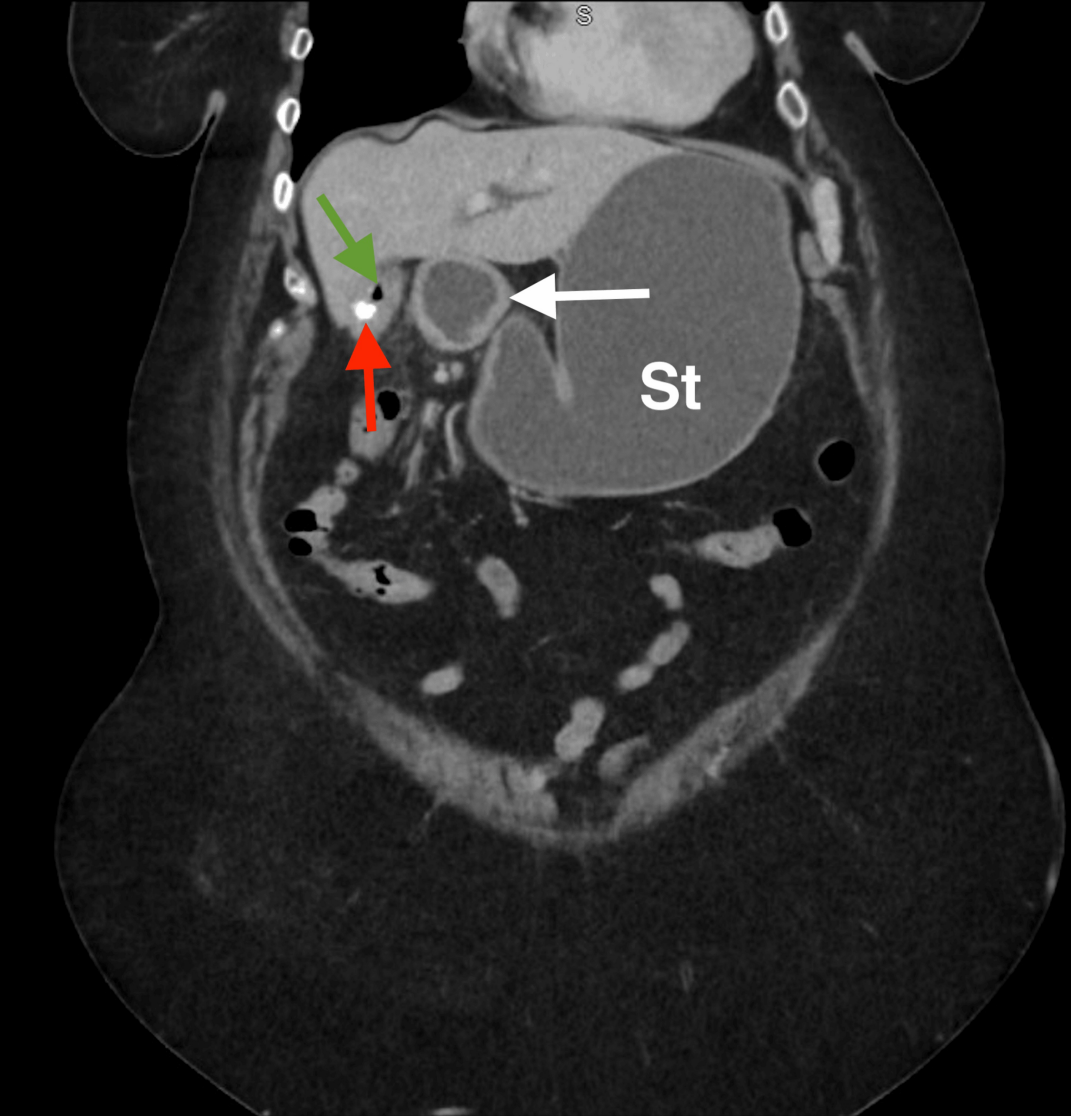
Coronal contrast-enhanced CT of the abdomen demonstrating Rigler’s triad in gallstone ileus. The green arrow indicates pneumobilia with air within the gallbladder/biliary system, the red arrow highlights gallstones within the gallbladder. The white arrow demonstrates the distended duodenal lumen, and the stomach (St) is markedly distended, consistent with distal duodenal obstruction.
